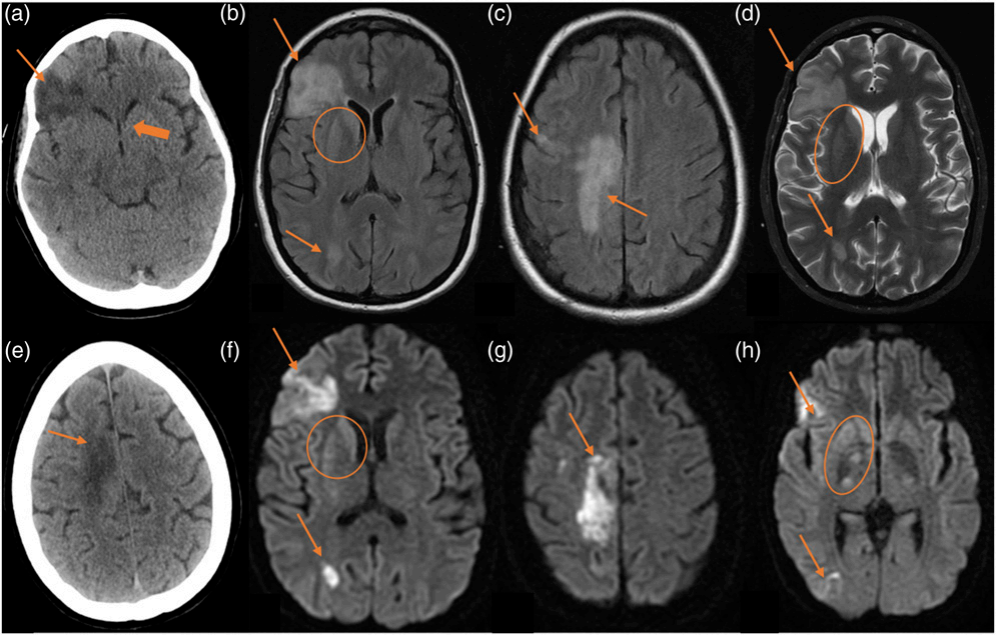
Multiple ischaemic strokes and anoxic encephalopathy. A 35-year-old woman with cystic fibrosis underwent double-LT. She suffered from severe haemodynamic compromise secondary to septic shock 5 days after the intervention. Figures: axial CT scan (a and e), axial MRI FLAIR sequence (b and c), axial MRI T2-weighted sequence (d) and axial MRI diffusion-weighted (f–h). CT scan reveals ischaemic strokes (arrows in a and b) and brain swelling with collapse of the ventricular system (thick arrow in a). There are large corticosubcortical hyperintensities on T2 and FLAIR (arrows in b–d), with restricted diffusion (arrows in f–h), in the right fronto-opercular, right parasagittal frontal and right occipito-parietal cortical regions. All of these hyperintensities are compatible with subacute ischaemic strokes. There are also hyperintensities on T2 and FLAIR with restricted diffusion (circles in b, d, f and h) in the right caudate and globus pallidus related to anoxic encephalopathy.
